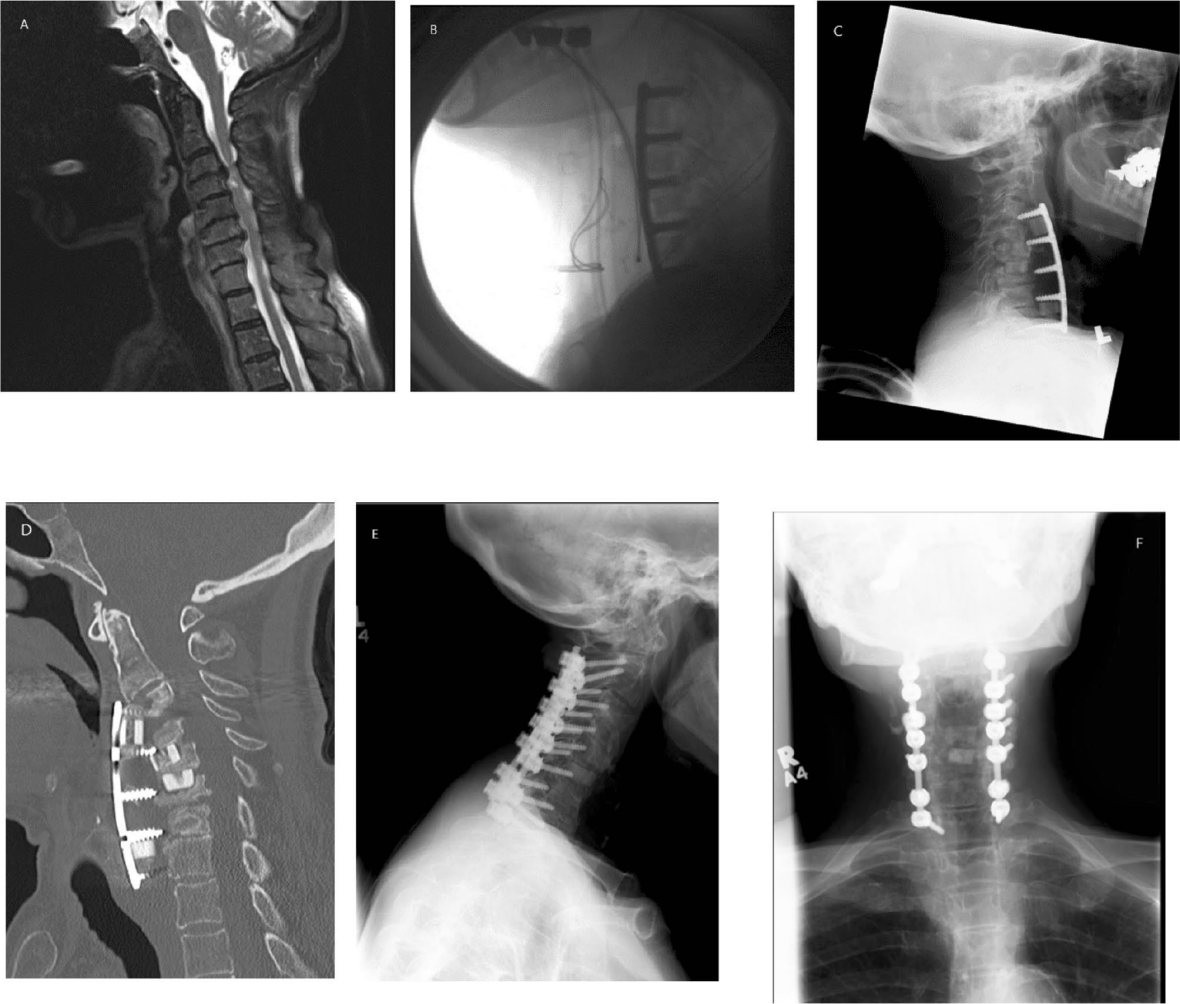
A 77-year-old female who presented with severe progressive myelopathy. A MRI scan of the cervical spine showed significant multilevel stenosis and cord compression (A). The patient underwent a four-level ACDF at the levels C3–C7 with good result (B). She was seen on a routine 8-week postoperative visit. The patient had no specific complaints and no swallowing dysfunction. She was improving neurologically. Lateral X-ray films showed complete dislodgement of the anterior hardware at all levels with the new onset of kyphosis (C,D). The patient underwent urgent revision with removal of previous anterior fixation hardware, repeat bone grafting C3–C7 and posterior C2–C5 decompression and C2–Th1 instrumented fusion (E,F). The ultimate outcome was excellent with significant resolution of symptoms.
